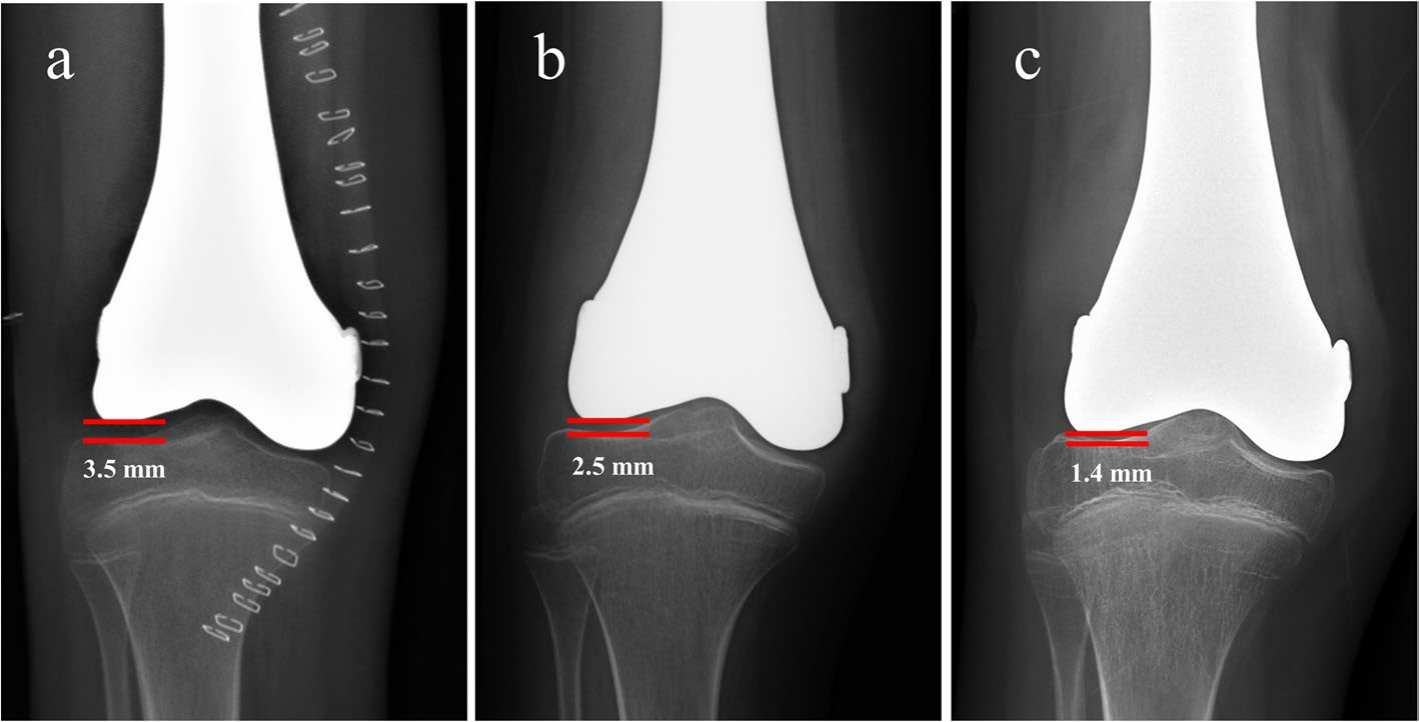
The radiograph of case 1 showing the progressing joint space narrowing. a After the surgery, joint space was 3.5 mm; b Ten months after the surgery, joint space was 2.5 mm; c 27 months after the surgery, joint space was 1.4 mm
